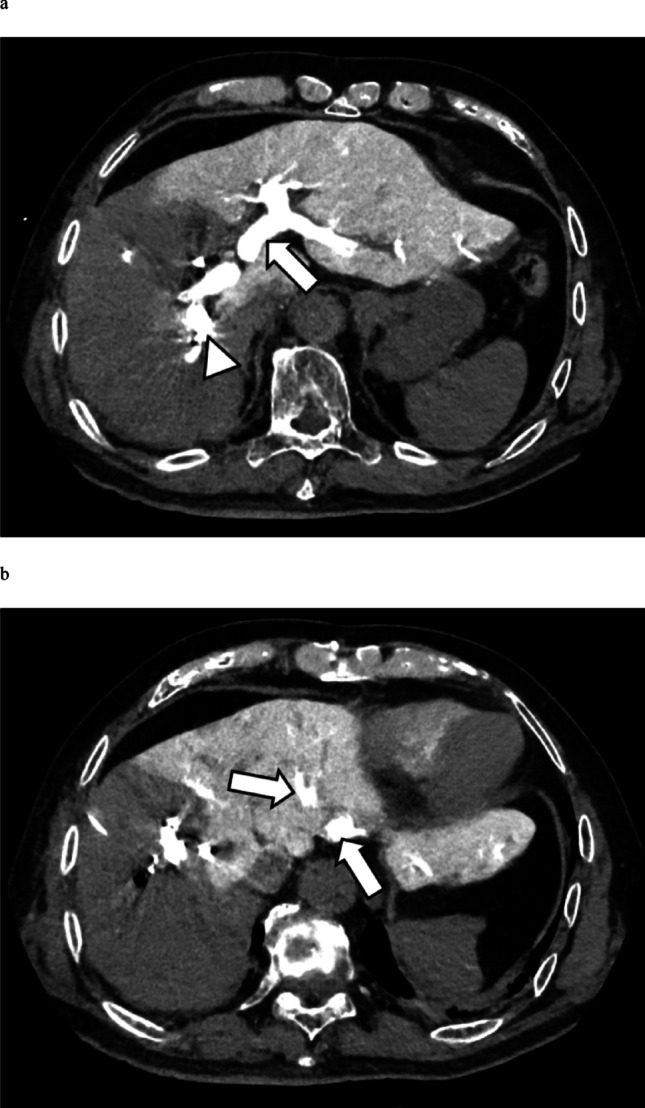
Immediately following PVE, contrast medium was injected via a catheter placed in the portal vein, and DECT imaging of the liver was performed. a Image acquired 6 s after contrast injection. Following embolization of the right portal vein branch, enhancement is confined to the left hepatic lobe and caudate lobe. The portal vein containing the injected contrast medium is clearly visualized (arrow). The arrowhead indicates the metallic coils. b Image acquired 15 s after contrast injection. While the contrast medium within the portal vein demonstrates washout, the hepatic veins (arrow) are distinctly visualized. DECT, dual-energy computed tomography; PVE, portal vein embolization
